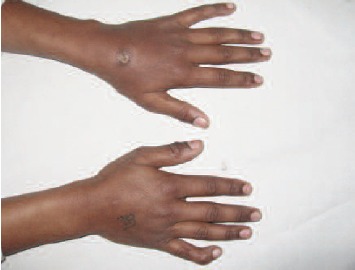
Fig. 2a: AP radiograph at presentation showing a lytic lesion in capitate. F2a:
